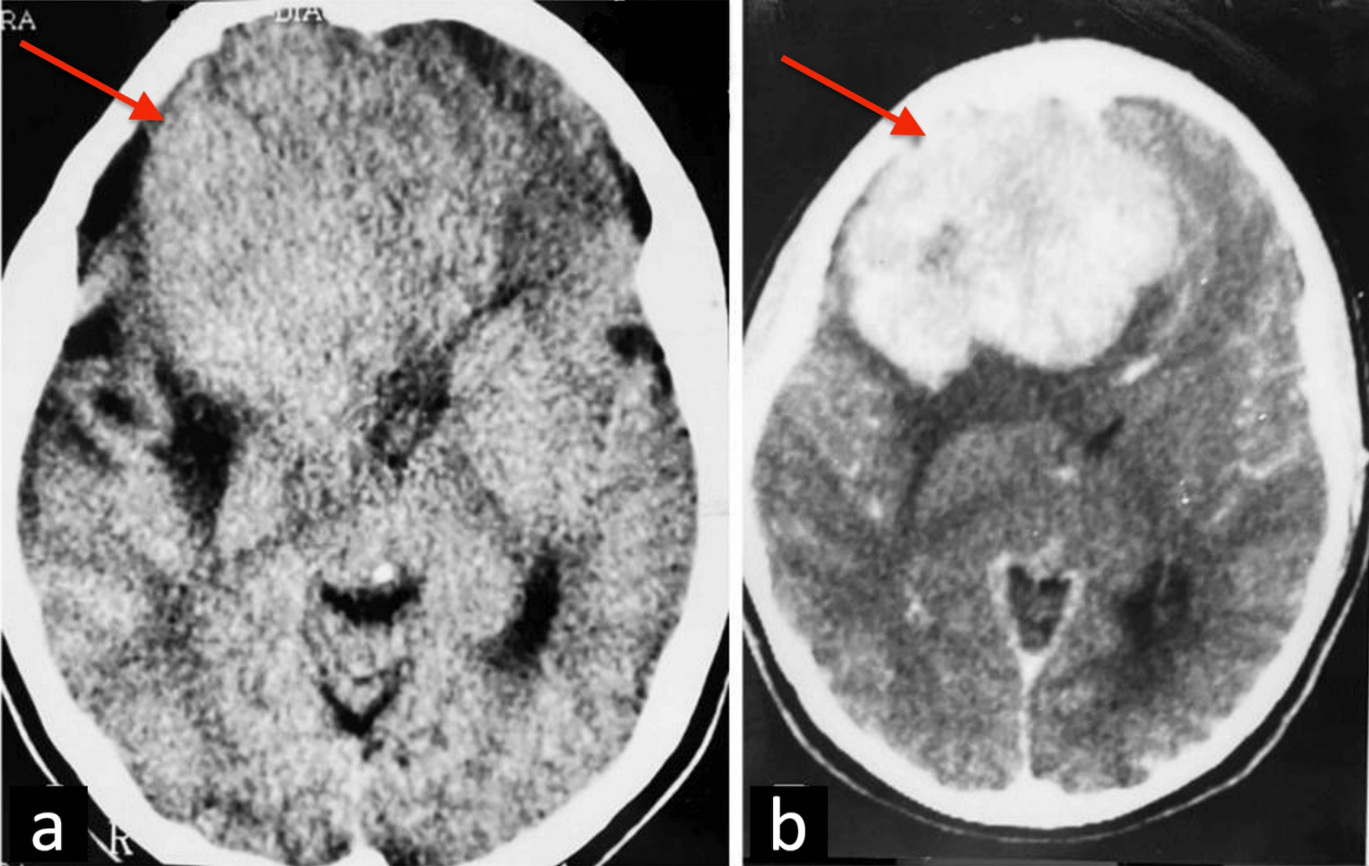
Brain CT findings a: brain CT scan revealed a large right frontal tumor (red arrow); b: with homogeneous contrast enhancement (red arrow).
